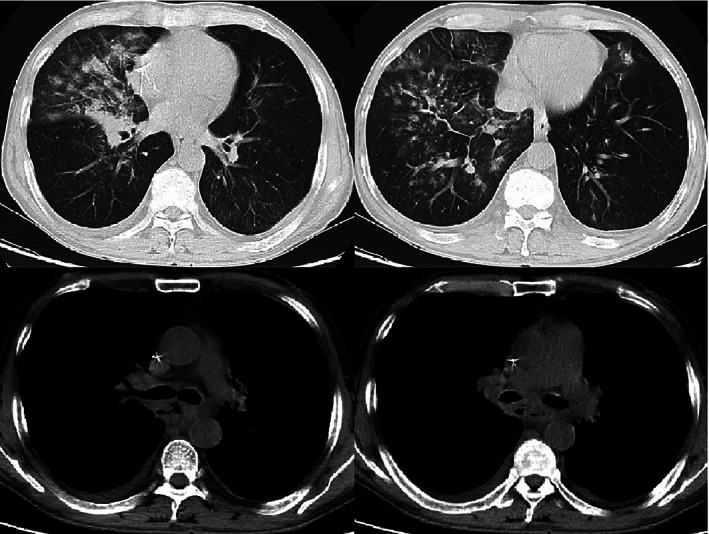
Representative CT images of pneumonia and tracheoesophageal fistula
